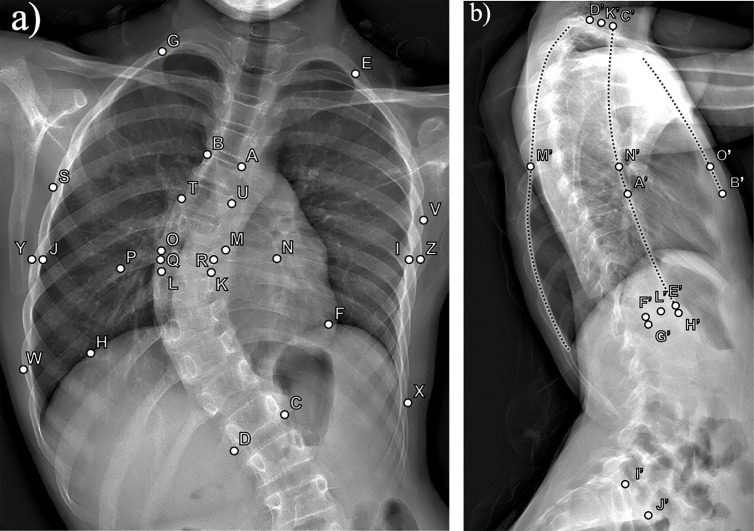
Anatomical landmark points used to define thoracospinal deformity parameters. Exemplar pair of a) frontal and b) sagittal plane radiographs with the anatomical landmark points used to calculate thoracospinal deformity parameters according to the formulas defined in Table 1.
